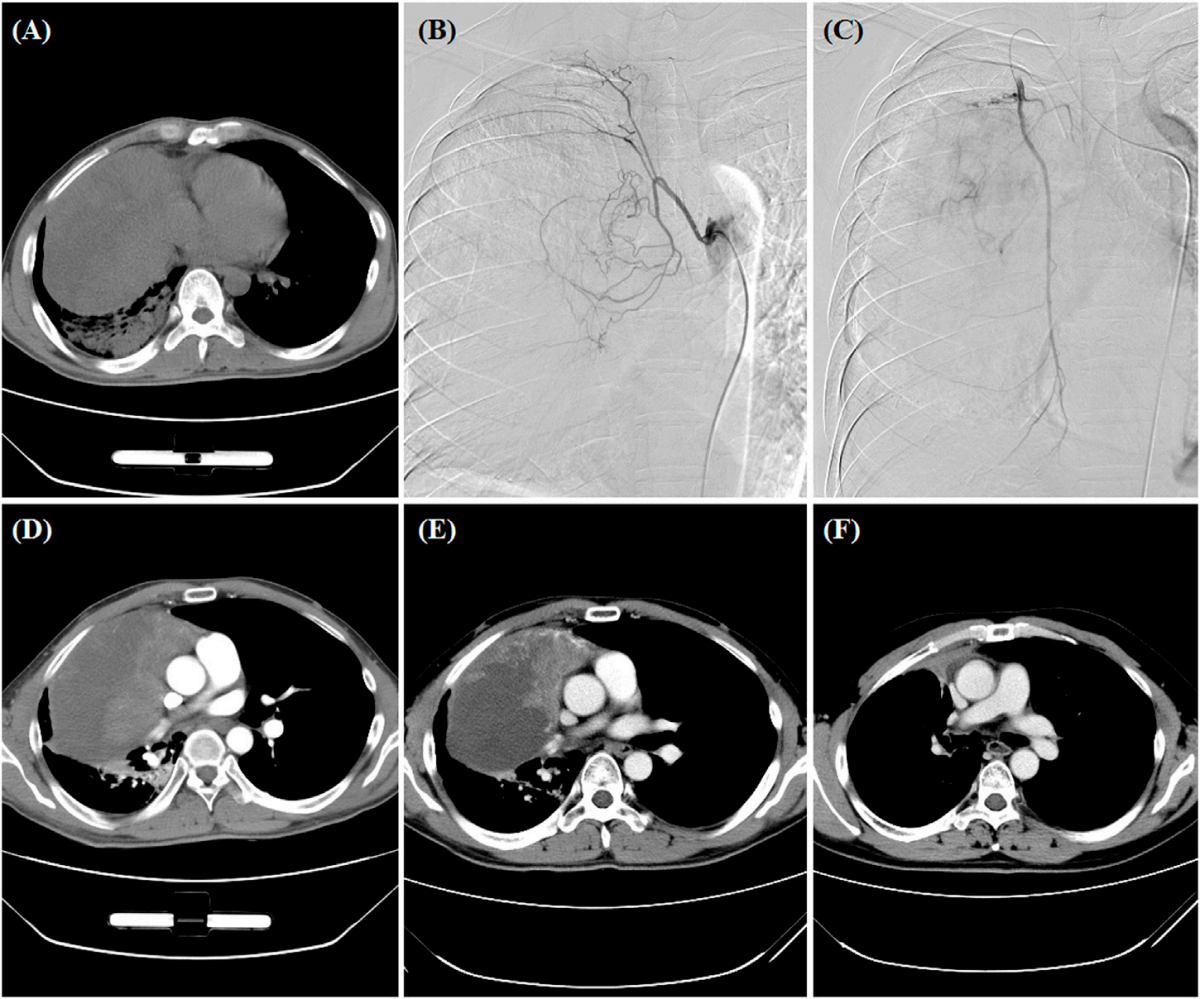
A 34-year man treated by DEB-TACE. (A) CT pre-procedure examination revealed a large tumor of the right lung. (B,C) The right bronchial artery and the right intercostal artery were the blood supply artery of the tumor. (D,E) The lung tumor was found to shrink at 1- and 3-month follow-up. (F) Surgical resection was performed after 3.6 months.
